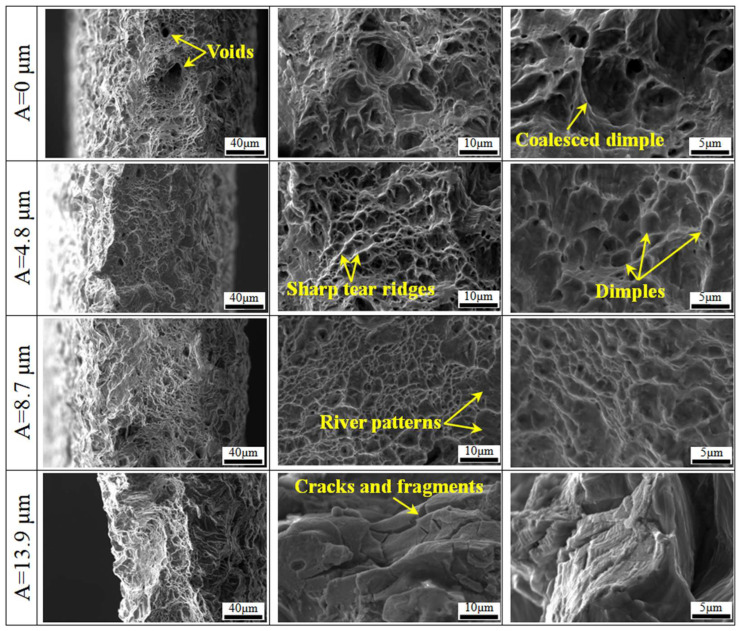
Fracture morphologies under various ultrasonic amplitudes.
